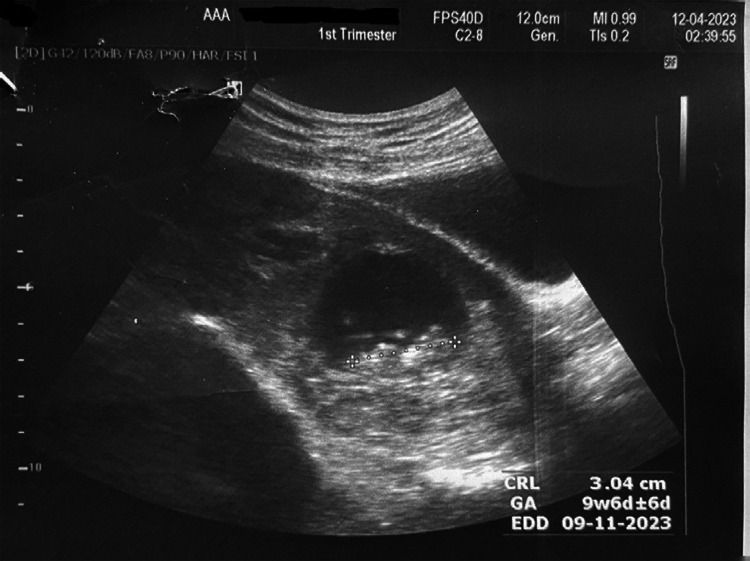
Ultrasonogram showing crown rump length.
